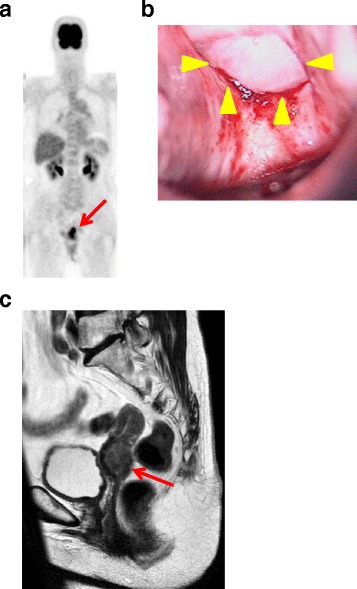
Imaging and physical examinations. a Positron emission tomography-computed tomography shows high 18F-fluorodeoxyglucose uptake consistent with a uterine tumor (*red arrow*). b Colposcopic view shows a solid white tumor on the posterior fornix (*yellow arrowheads*). c Pelvic magnetic resonance image of a T2-enhanced sagittal section showing an irregularly enlarged uterine cervical tumor (*red arrow*)
